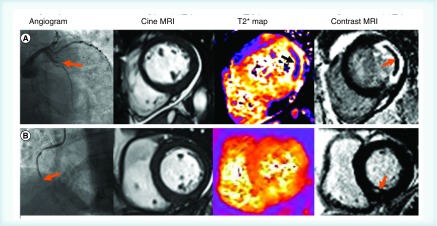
Two patients with acute ST-elevation myocardial infarction who had cardiac MRI 2 days post-event including T2* mapping. Angiography in patient A revealed an acutely occluded large obtuse marginal branch (orange arrow). Contrast MRI revealed a lateral infarct with significant microvascular obstruction (orange arrow). This corresponded to an area of intramyocardial hemorrhage as revealed by T2* mapping (black arrow). Patient B suffered an acute occlusion of the mid segment of the right coronary artery (orange arrow). Contrast MRI revealed a small infarct territory (orange arrow) with no intramyocardial hemorrhage visible on the T2* map.
